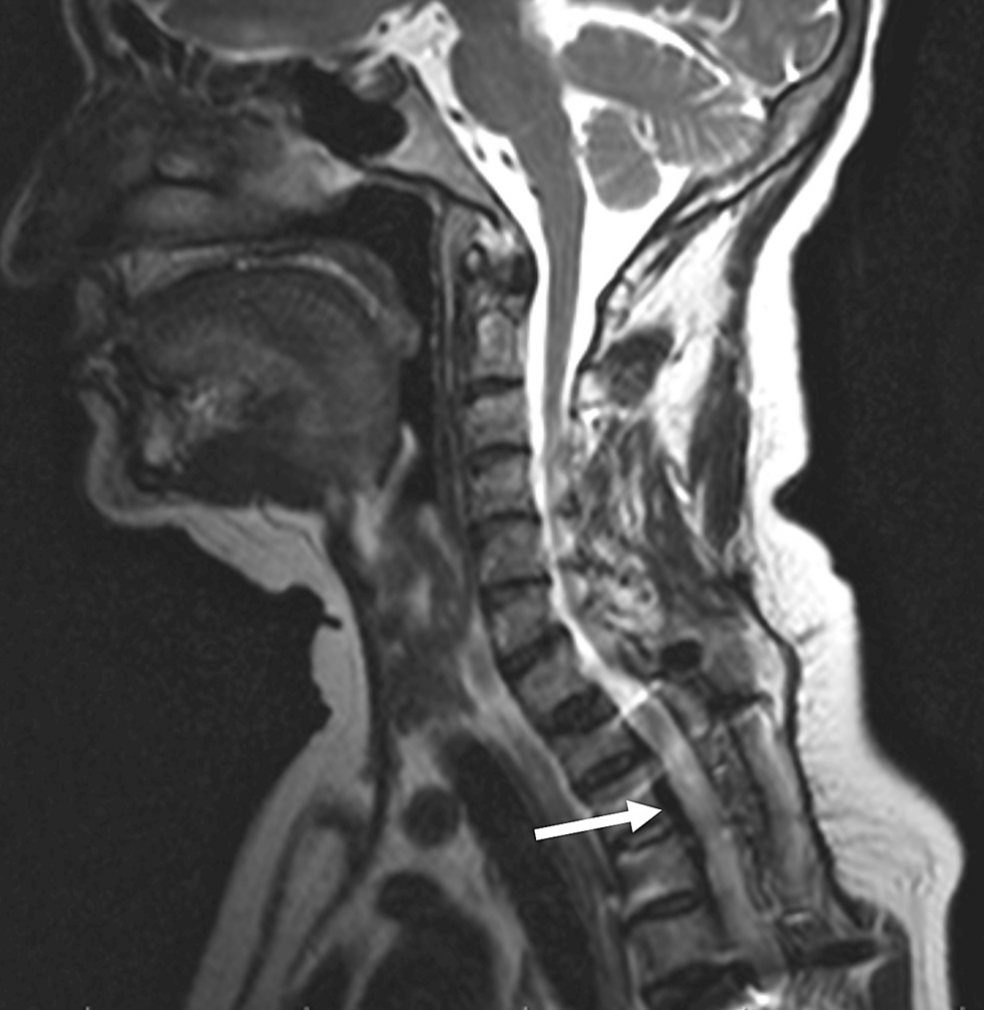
Postoperative MRI (T2 sagittal) showing near-complete resolution of the extradural mass lesion (arrow).
